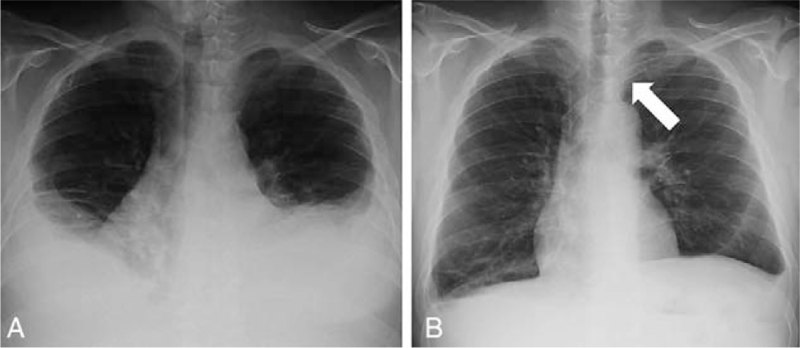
(A) Chest X-ray performed at the admission showing a bilateral pleural effusion. (B) Chest X-ray performed 1 month after the intervention showing near resolution of pleural effusions. The stent in the left brachiocephalic vein is clearly visible (arrow).
